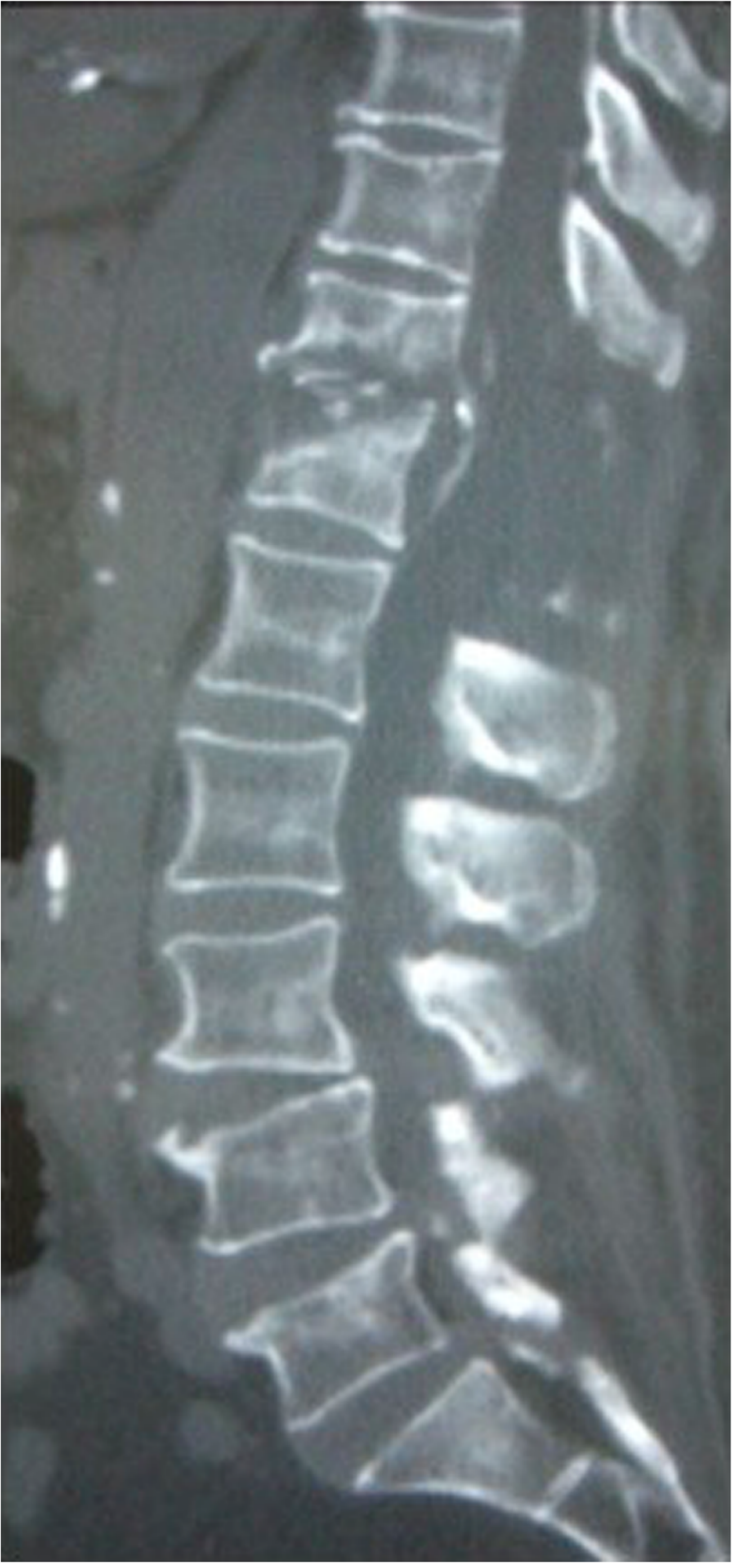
Computed tomography at 6 weeks after operation. T12 vertebral bone destruction is seen.
